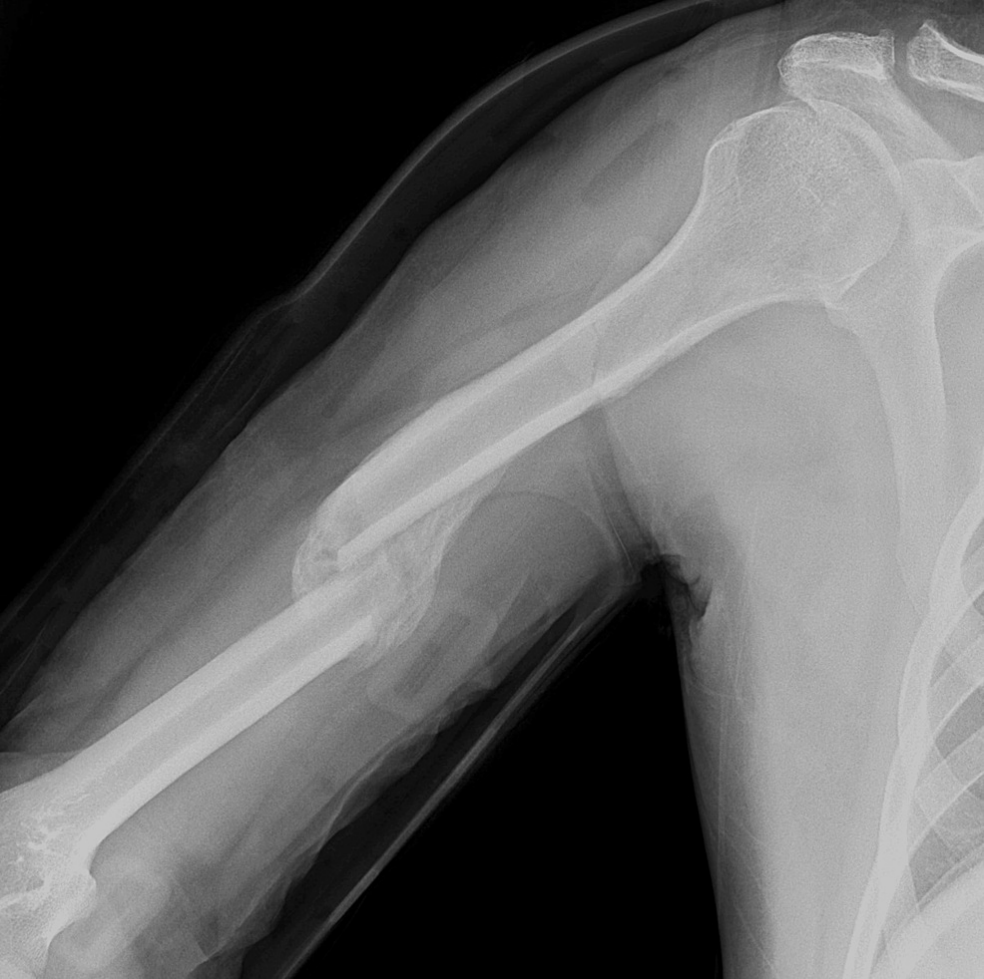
Plain radiograph shows a right humerus callus 32 days after the accident
